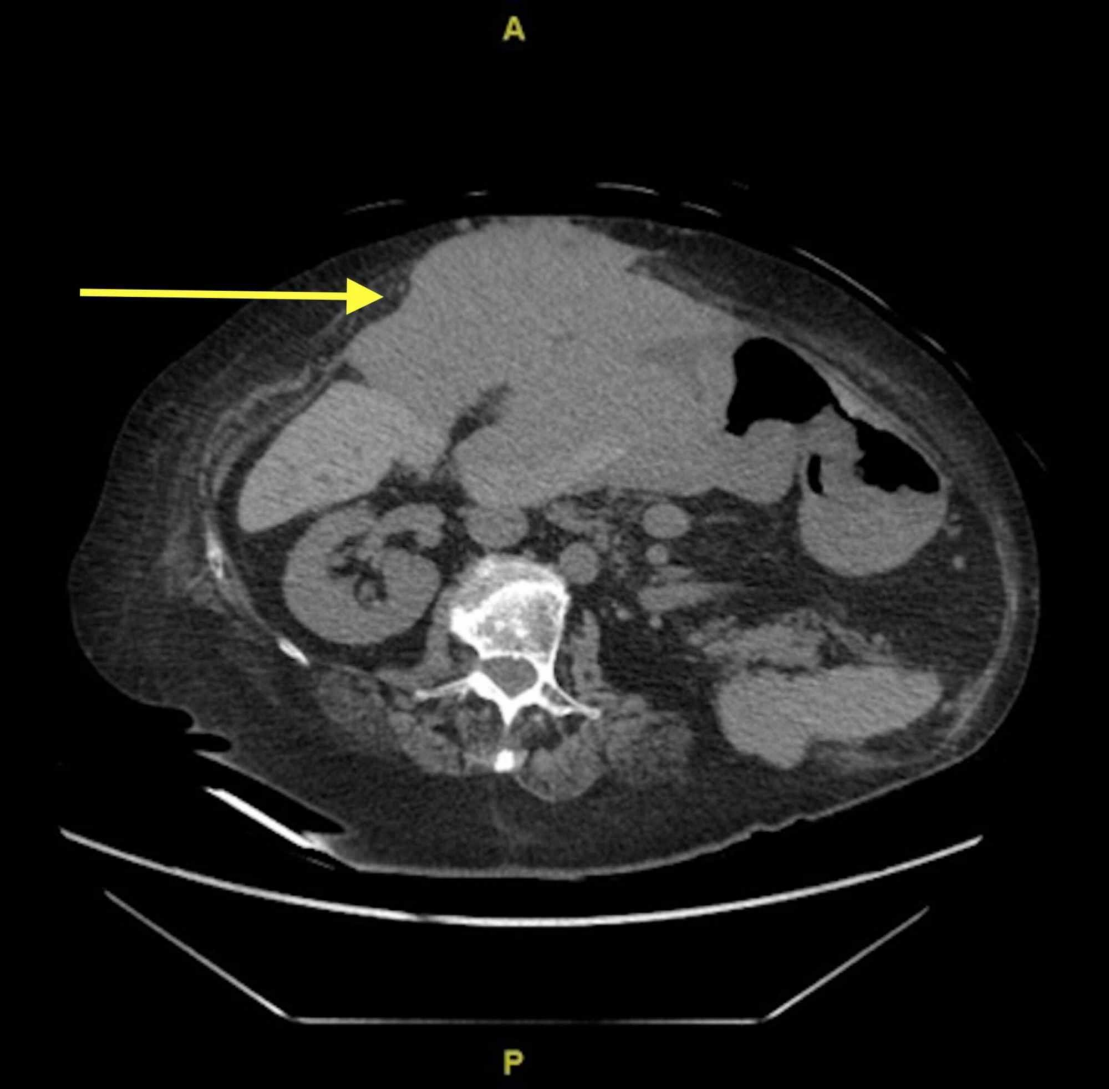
Transverse CT with IV contrast showing a soft tissue mass abutting the anterior liver, infiltrating the anterior abdominal wall and involving the stomach
